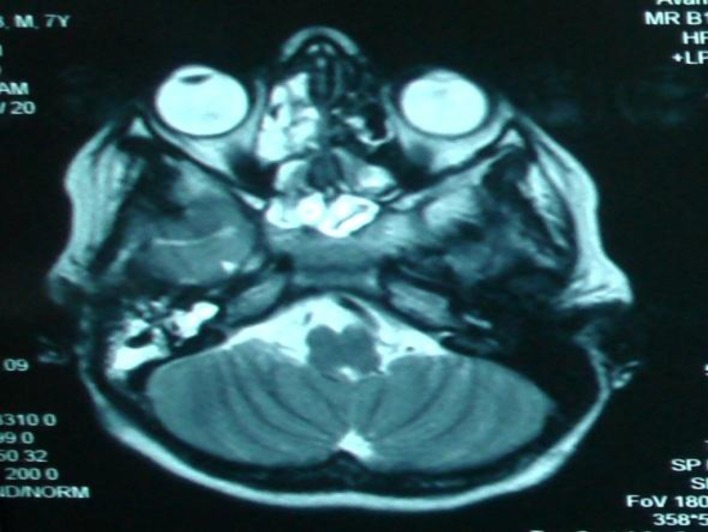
T2-MRI showing fluid in the ear and sinuses
